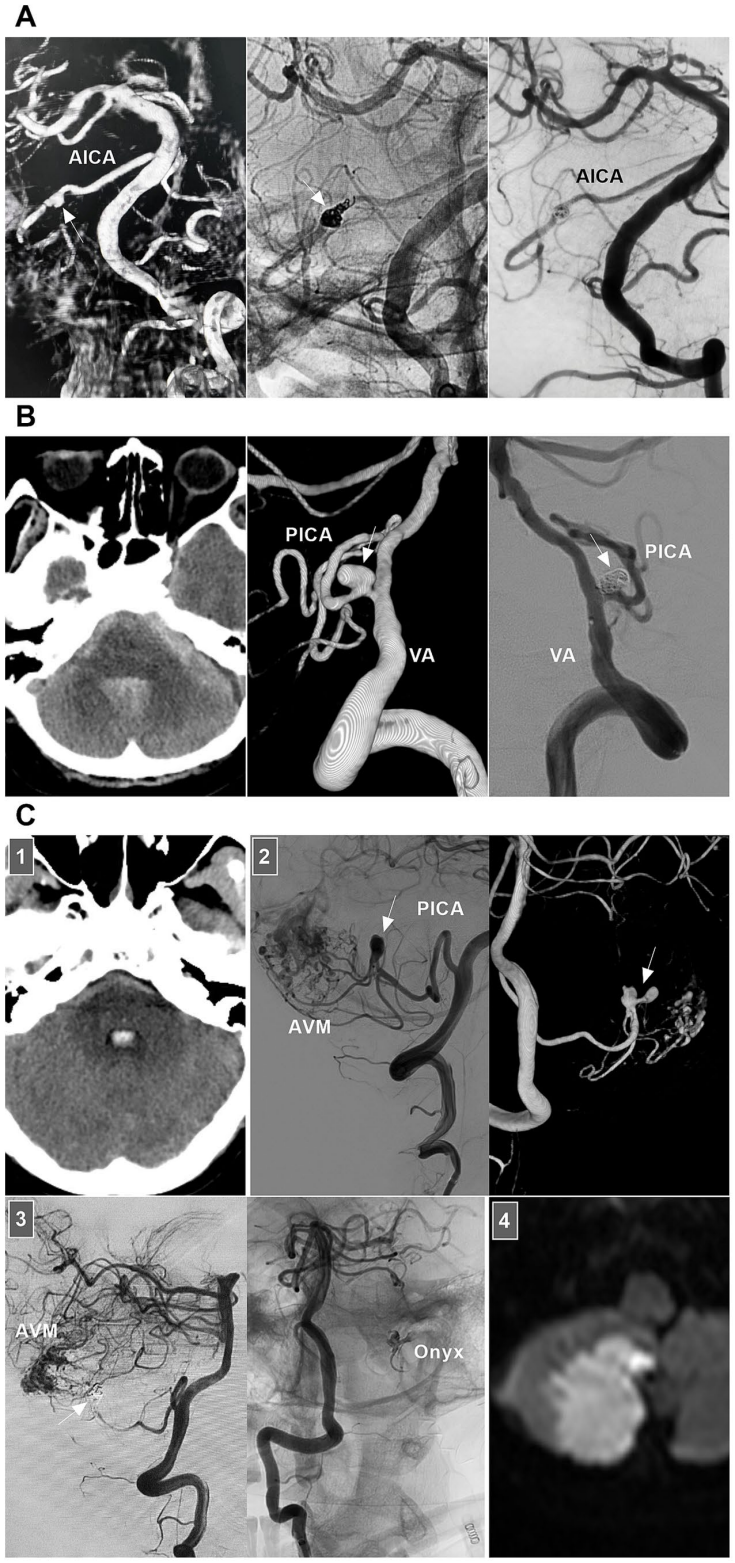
EVT of AICA and PICA dissections. (A) Left panel: DSA showing a ruptured AICA dissection (arrow). Middle panel: Unsubtracted DSA showing the dissection (arrow) was coiled. Right panel: DSA showing the AICA was patent, and chronic occlusion was worthy to expected. (B) Left panel: CT showing SAH. Middle panel: DSA showing a ruptured PCA sidewall dissecting aneurysm (arrow). Right panel: DSA showing the aneurysms (arrow) was coiled selectively. (C) Panel 1: CT showing the fourth ventricle hemorrhage. Panel 2: DSA (left panel) and its reconstructive image (right panel) showing an AVM that supplied by PICA with flow-related dissecting aneurysms (arrows). Panel 3: DSA (left panel) showing the aneurysms were embolized (arrow), unsubtracted DSA (right panel) showing the Onyx in the aneurysm. Panel 4: Diffuse weighted image of magnetic resonance showing asymptomatic acute ischemia of cerebellar hemisphere. AICA, anterior inferior cerebellar artery; AVM, arteriovenous malformation; CT, computed tomography; DSA, digital subtraction angiography; EVT, endovascular treatment; PICA, posterior inferior cerebellar artery; SAH, subarachnoid hemorrhage; VA, vertebral artery.
